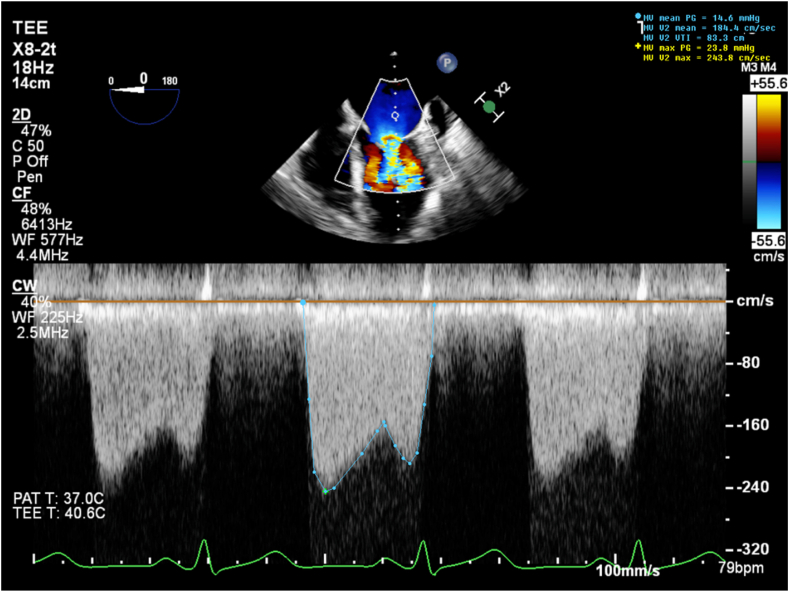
Two-dimensional TEE, mid-esophageal long-axis (0°) diastolic view with continuous-wave Doppler cursor positioned across the MV, demonstrates an abnormal spectral Doppler profile with severely elevated transmitral velocities consistent with a mean diastolic gradient of 15 mm Hg at 79 bpm and severe MS.
